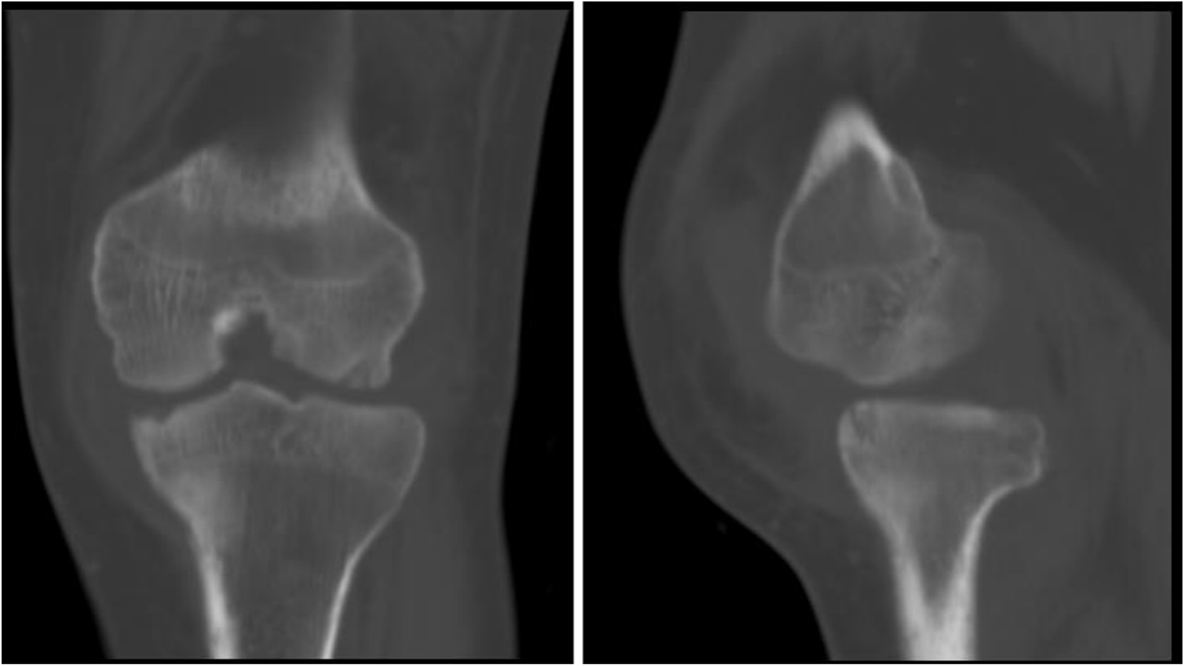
CT (anteroposterior and lateral): severe joint destruction and central and peripheral erosions
